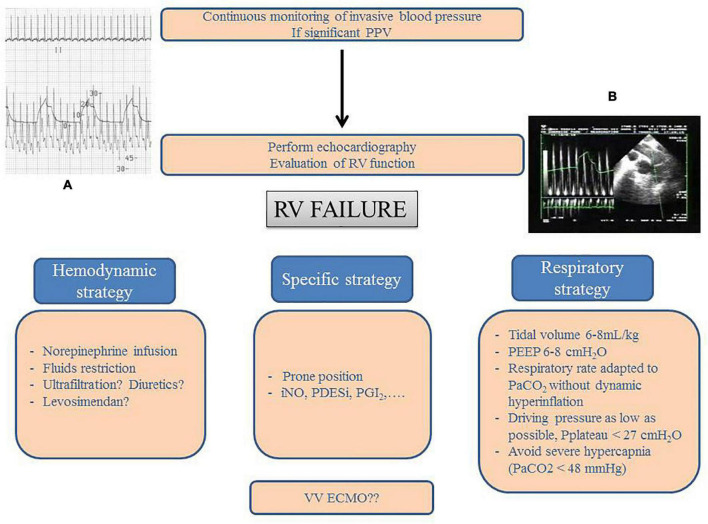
Right ventricle protective strategy. Principles for detection and management of right ventricular (RV) failure in patients with ARDS. This should combine invasive blood pressure monitoring and echocardiography. In the case of significant pulse pressure variation (PPV, Panel A), critical care echocardiography must be performed. It usually demonstrates cyclic decrease in RV outflow at each insufflation (Panel B on an upper esophageal view with pulsed wave Doppler into the main pulmonary artery) with either isolated RV dilatation or acute cor pulmonale. Management is based on three different strategies: hemodynamic, respiratory, and specific. VV ECMO can be considered in the case of persistent RV failure. PEEP, positive end-expiratory pressure; iNO, inhaled nitric oxide; PDESi, phosphodiesterase type 5 inhibitor; PGI_2_, prostaglandin I_2_; VV ECMO, veno-venous extracorporeal membrane oxygenation.
